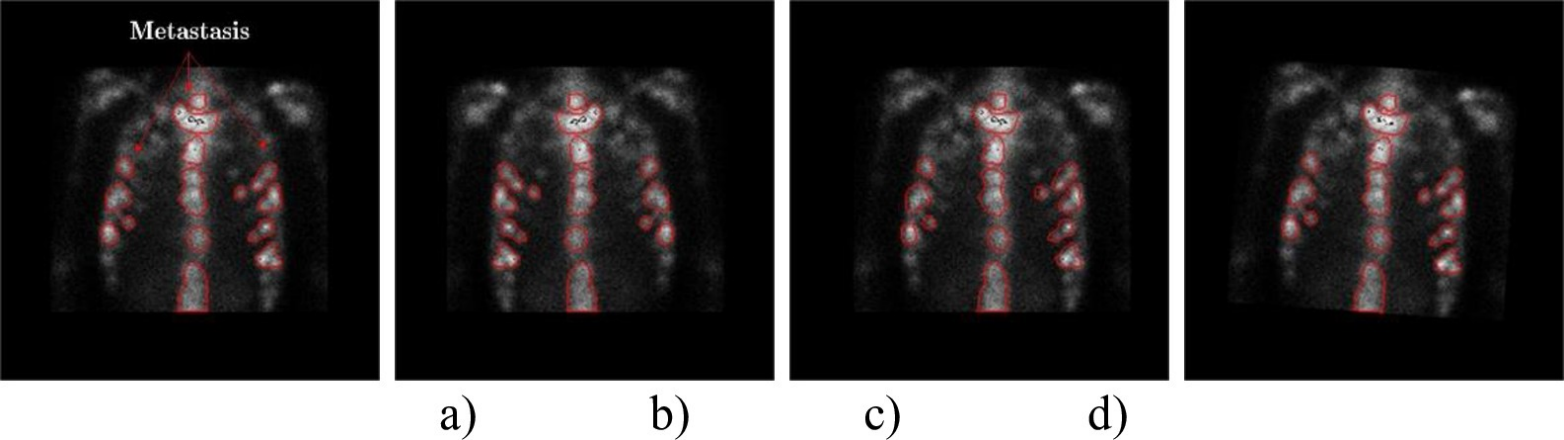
An example of preprocessing thorax bone SPECT image. a) The original thorax bone SPECT image; b) The horizontally mirrored image; c) The horizontally translated image by + 6 pixels; and d) The rotated image by +5°.
